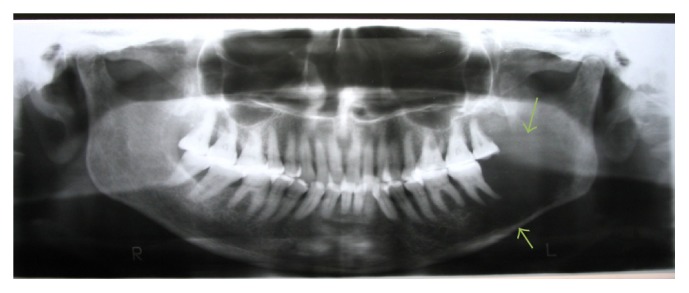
Preoperative orthopantomogram (arrow) showing diffuse irregular multilocular radiolucency in the left side of the lower jaw extending from parasymphysis region to the ramus of the mandible.
